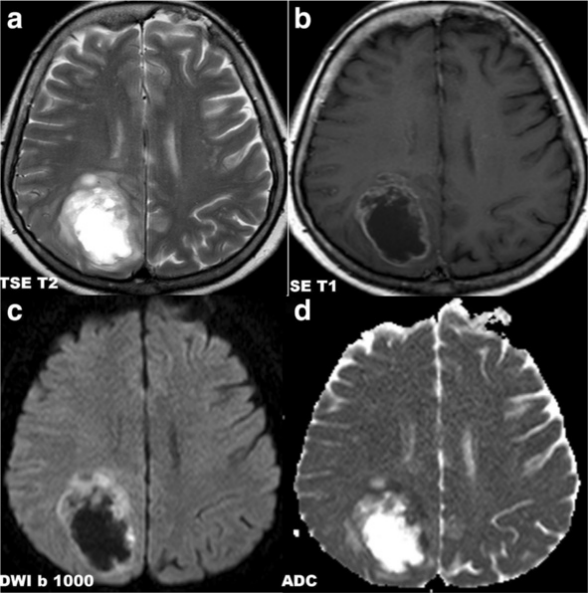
a) FSE T2 WI, axial scan b) SE T1 WI after intravenous gadolinium contrast administration, axial scan c) DWI, b=1000, axial scan d) DWI, ADC map, axial scan
